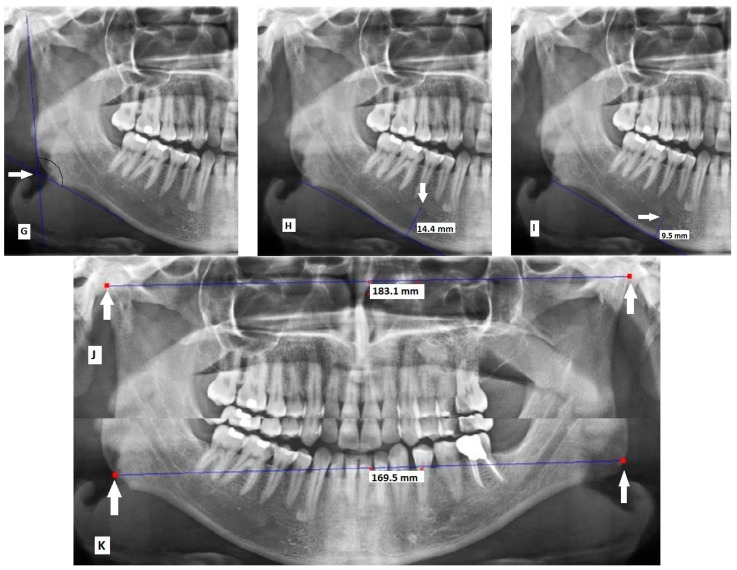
Measurements made on panoramic radiographs: (A) maximum ramus width; (B) minimum ramus width; (C) condylar height; (D) coronoid height; (E) antegonial angle; (F) antegonial depth; (G) gonial angle; (H) distance between the superior border of the mental foreman and the inferior border of the ramus; (I) distance between the inferior border of the mental foramen and the inferior border of the ramus; (J) bicondylar breadth; and (K) bigonial breadth.
